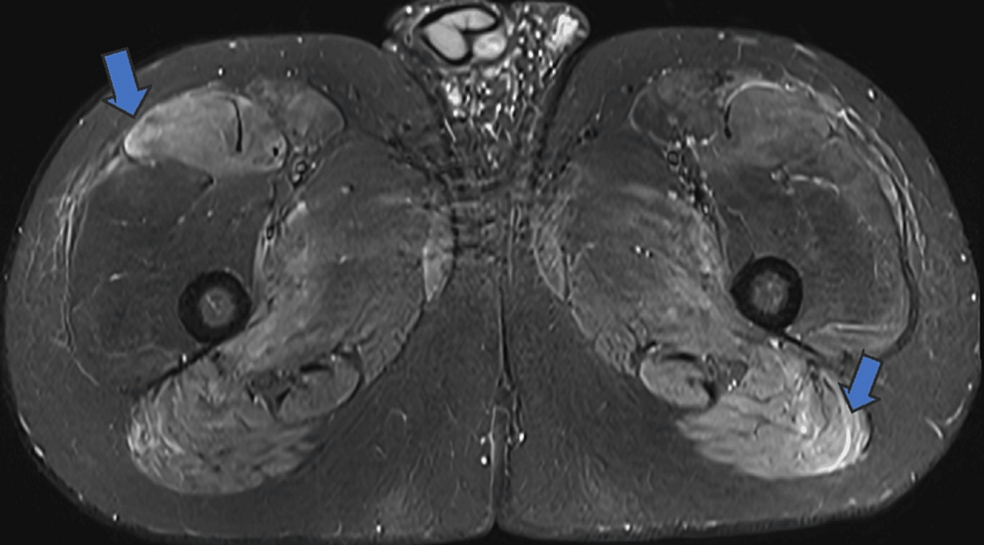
MRI shows bilateral high signal intensity, suggestive of acute myositis (blue arrows).
